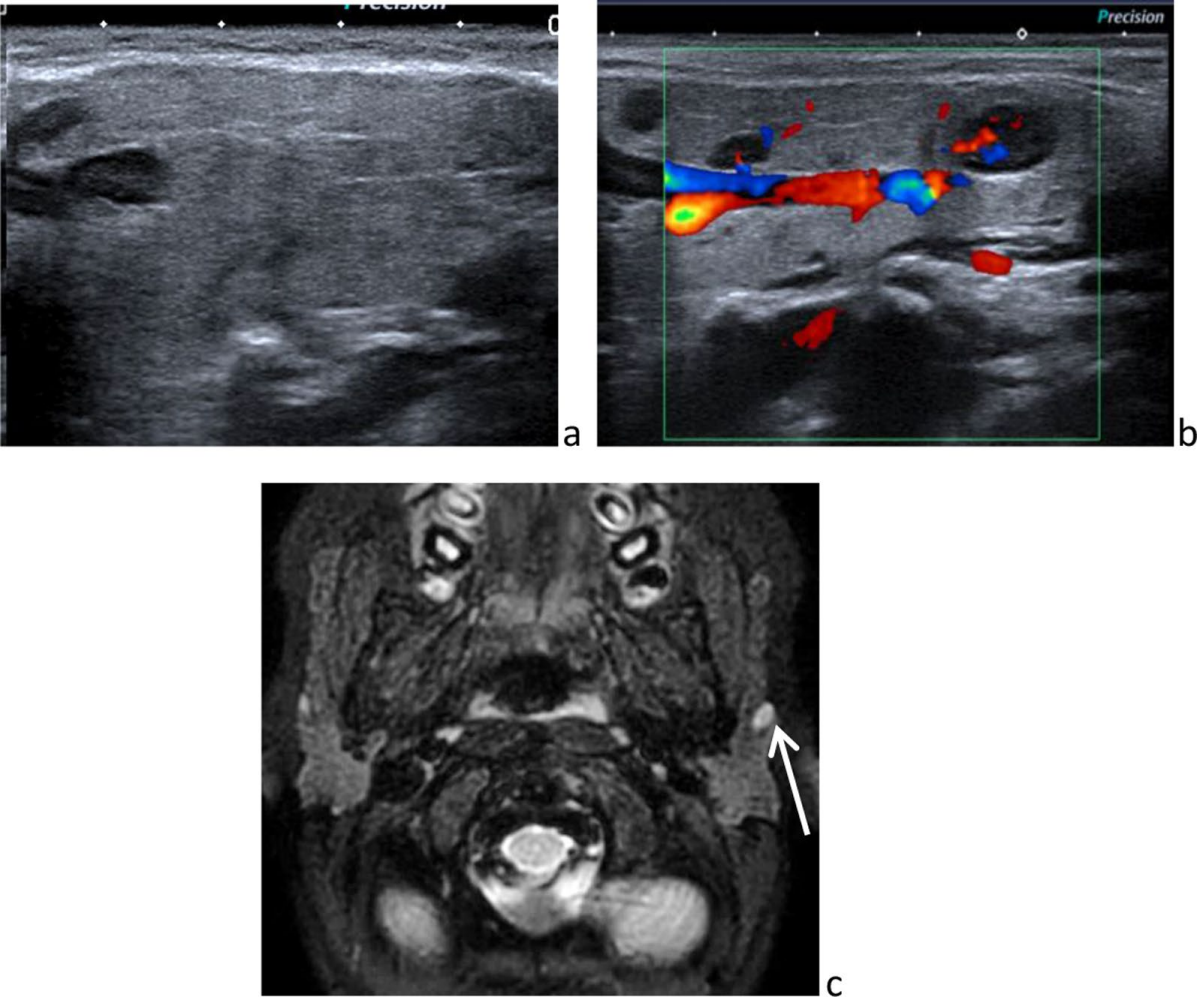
Intraparotid normal lymph nodes on sonography (a), color-Doppler sonography (b) and axial fat sat *T*_2_-weighted image (c)
